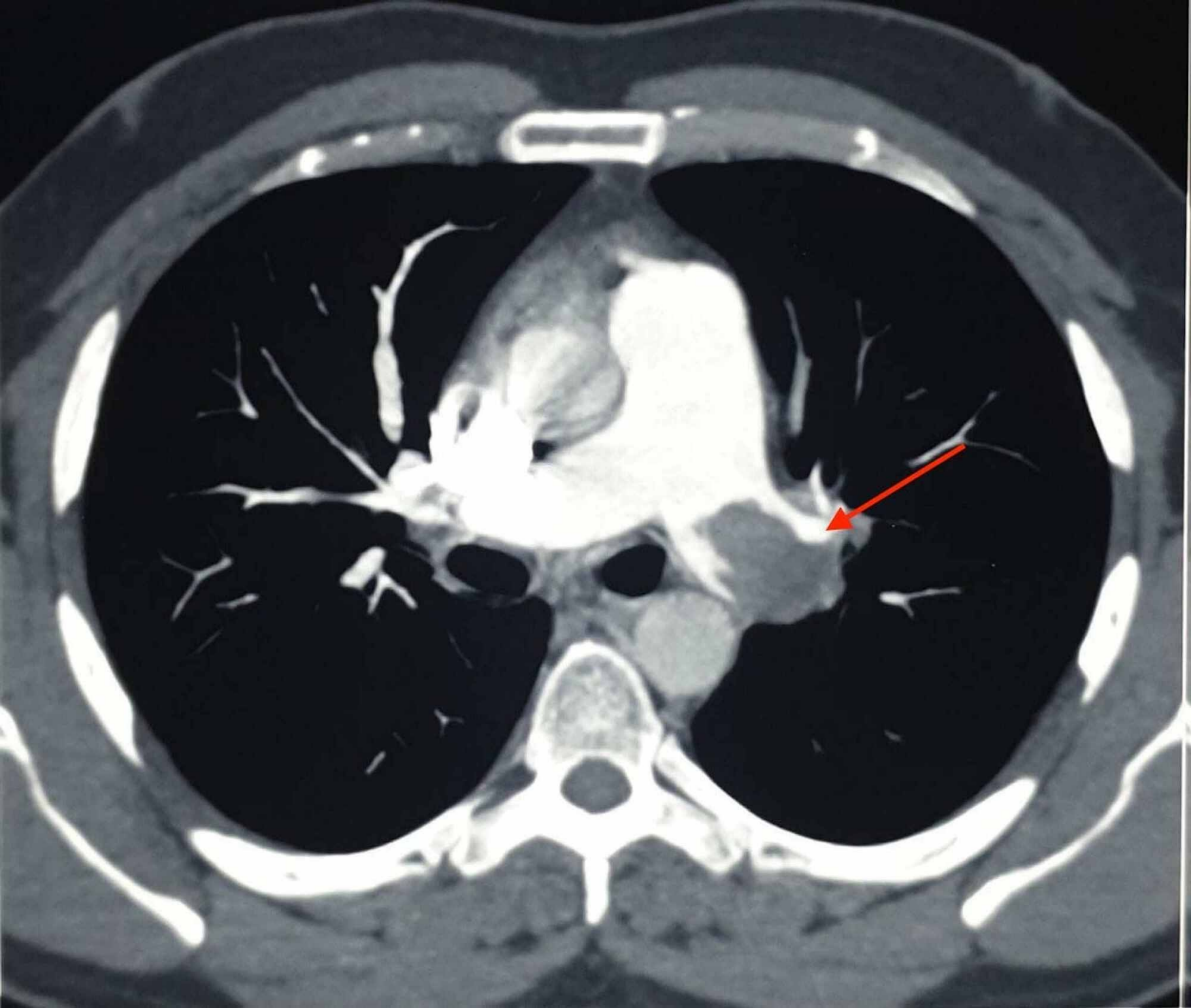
Axial section of CTPA scan showing a large hypodense filling defect in the left main pulmonary artery. CTPA - Computed tomography pulmonary angiogram
